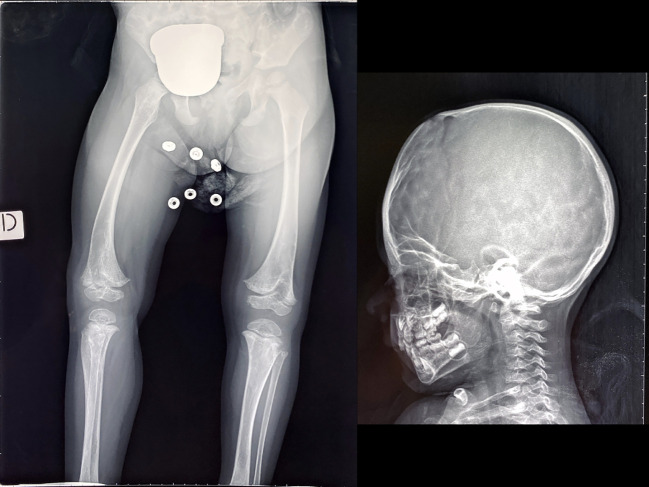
The radiographs of the proband from Family 1 showed subchondral areas of radiolucency in the distal femoral metaphysis, accompanied by irregularities in the metaphysis and generalized bone demineralization. The cranial vault exhibited marked mineralization defects with a distinctive "beaten copper" appearance.
